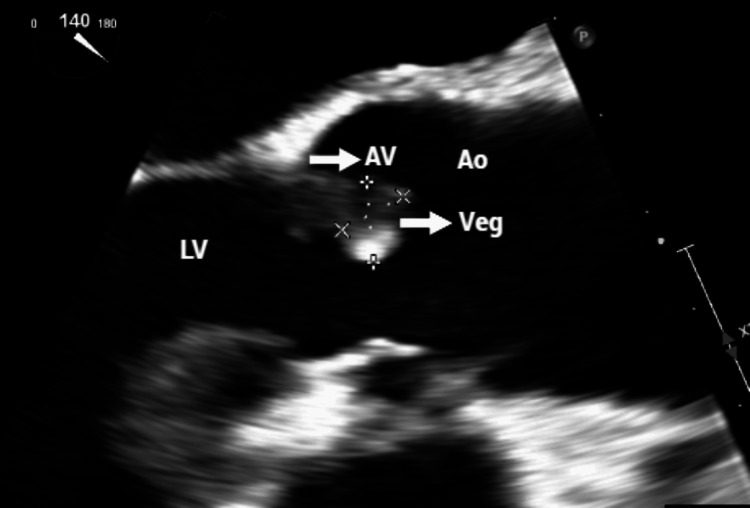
Transoesophageal echocardiogram demonstrating a mobile structure on the left coronary cusp of the aortic valve (AV) measuring 0.5 × 0.4 cm, consistent with vegetation (Veg). No abscess was identified. Ao: ascending aorta; LV: left ventricle
